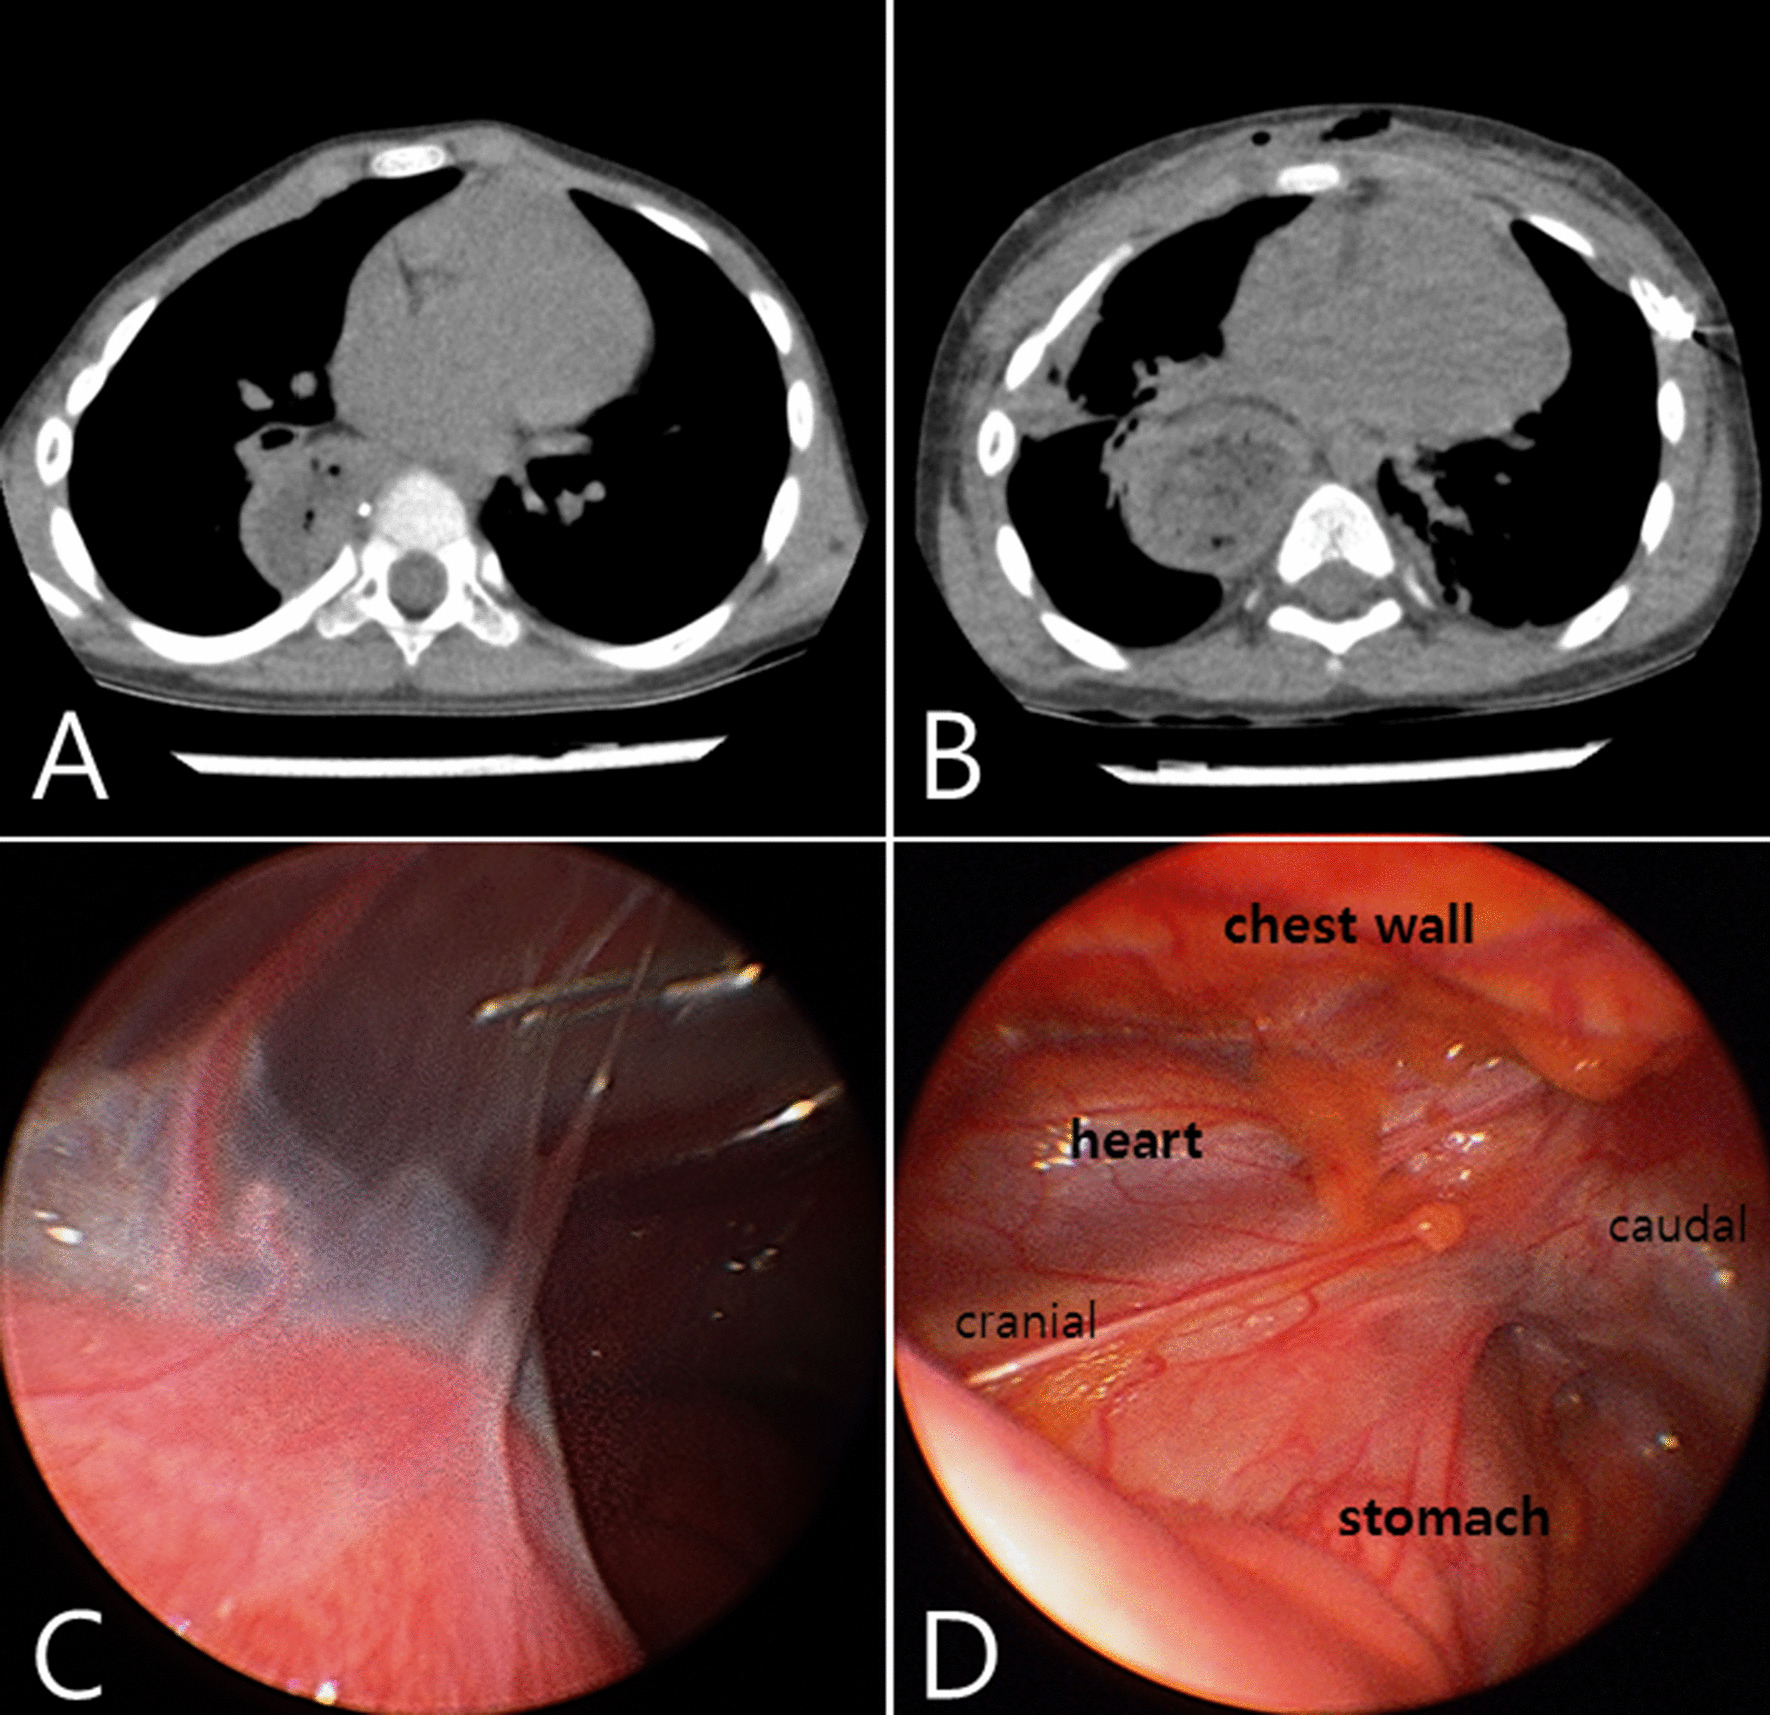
A Preoperative computed tomography (CT) showing depression of the right anterior chest wall with sternal protrusion and previously operated stomach in right side mediastinum. B Postoperative CT after insertion of two pectus bars and polyester suture. C A 2-mm needlescope showing localized pleural adhesions along the previous thoracotomy site. D Depressed right costal cartilages and stomach
